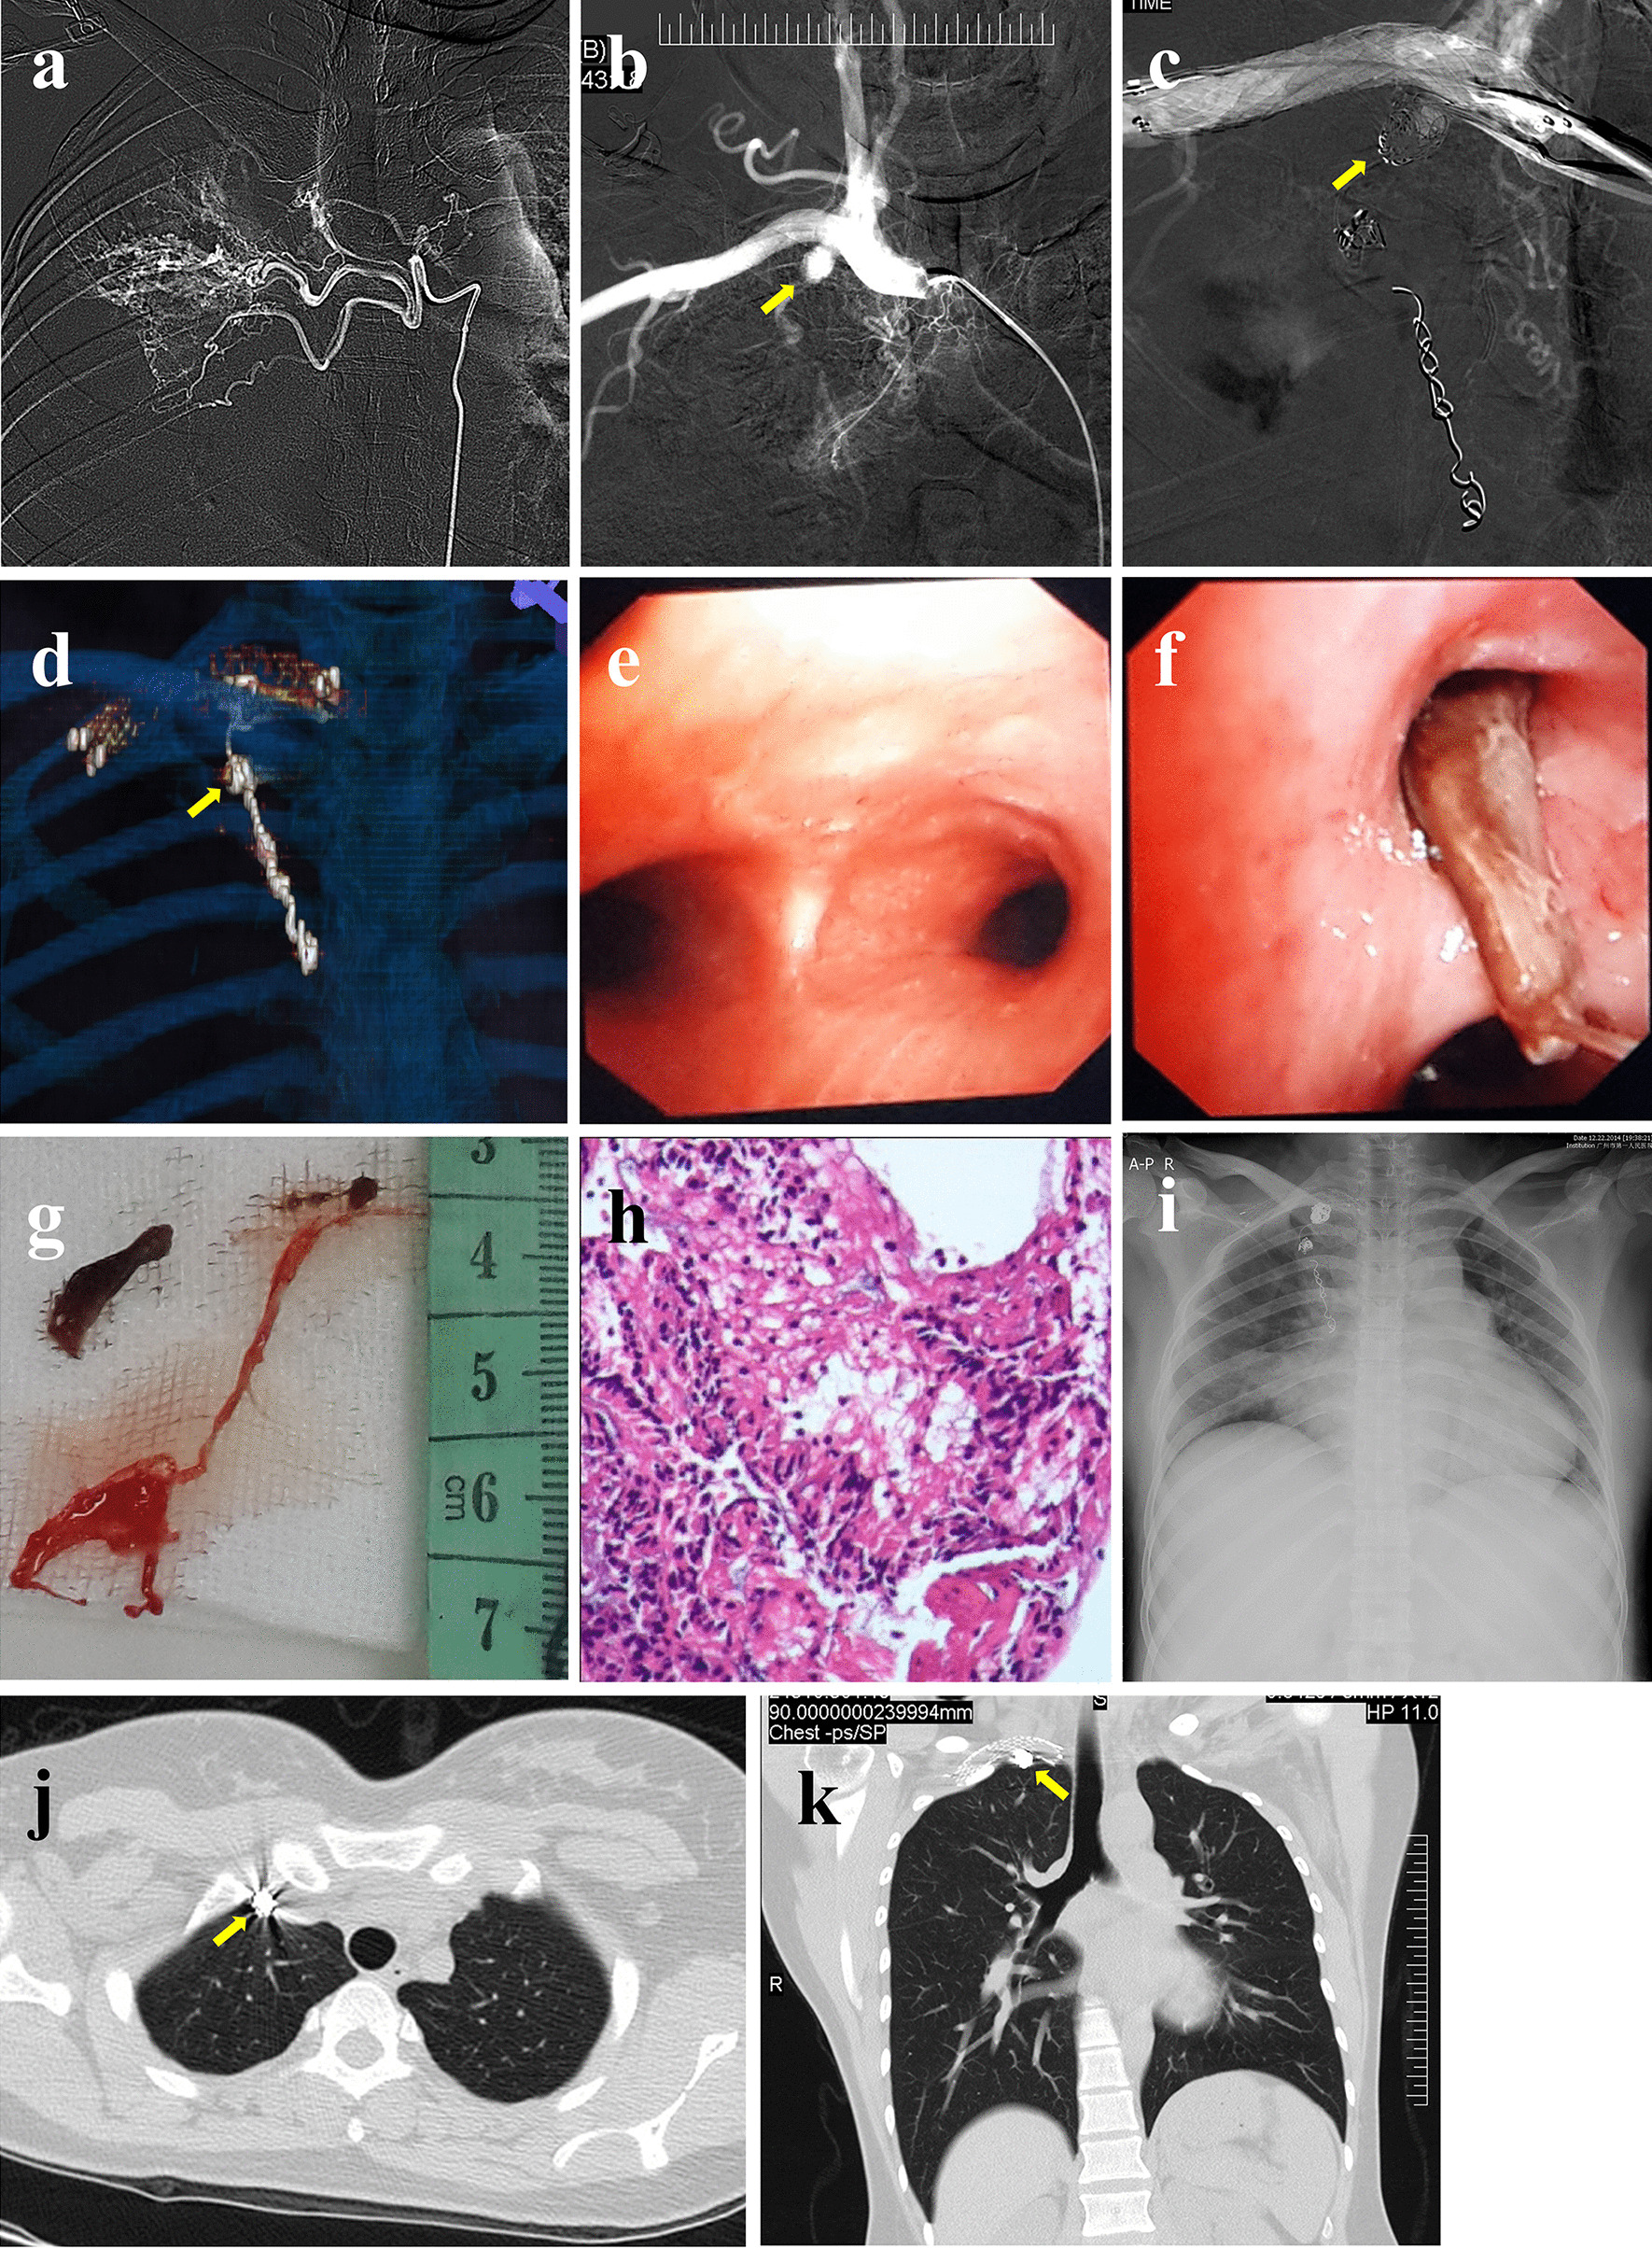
Arteriogram revealing the tortuosity, dilation and hypertrophy of the right bronchial arteries (a). Aneurysms (yellow arrow) of the internal thoracic artery before (b) and after (c) metal coil embolization. Three-dimensional image shows aneurysms embolized by a metal coil (yellow arrow) (d). Bronchoscopy was used to remove blood clots (g), as confirmed by pathological examination (h), that were blocking the right-sided mainstem bronchus (e) and upper lobe bronchus (f). Chest radiograph indicates reexpansion of the right lung after removal of the clots (i). Follow-up CT shows the metal coil (yellow arrow) and the right lung returned to normal (j axial views; k coronal views)
